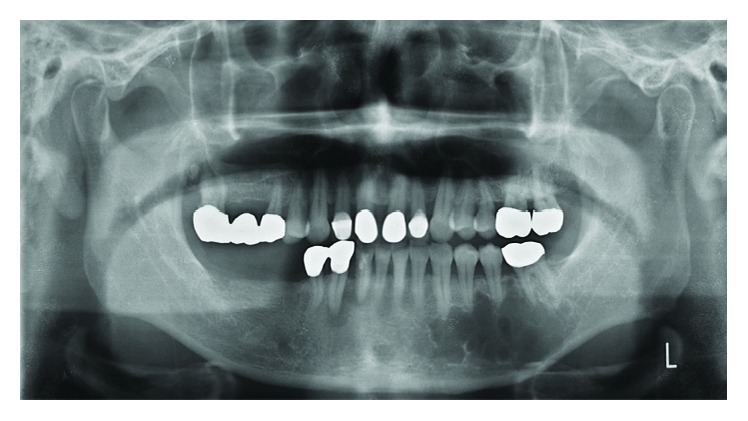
Panoramic dental radiography before surgery. The image shows extensive multilocular radiolucent area with imprecise borders and “soap bubble appearance”.
